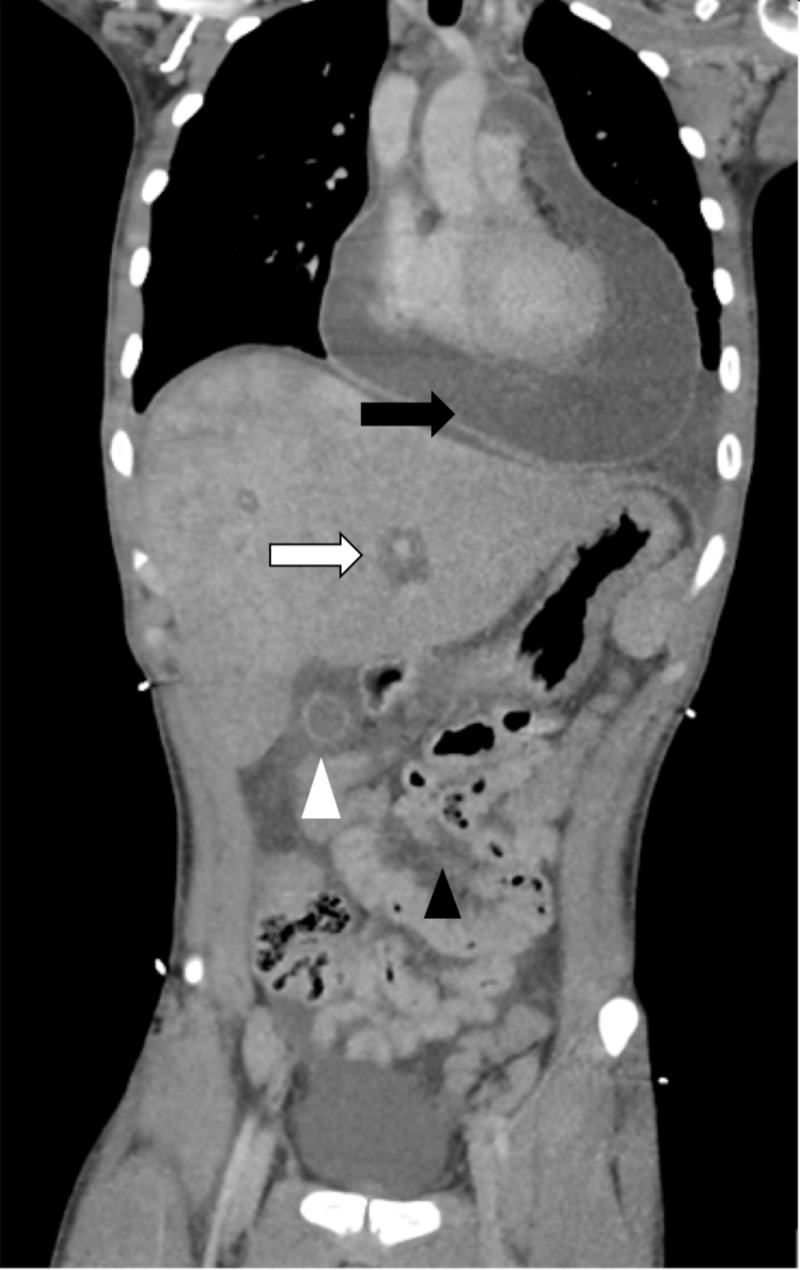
Computed tomography (CT) imaging showing the pericardial effusion (black arrow), ascites (black arrowhead), hepatic congestion (white arrow), and gallbladder wall thickening (white arrowhead)
